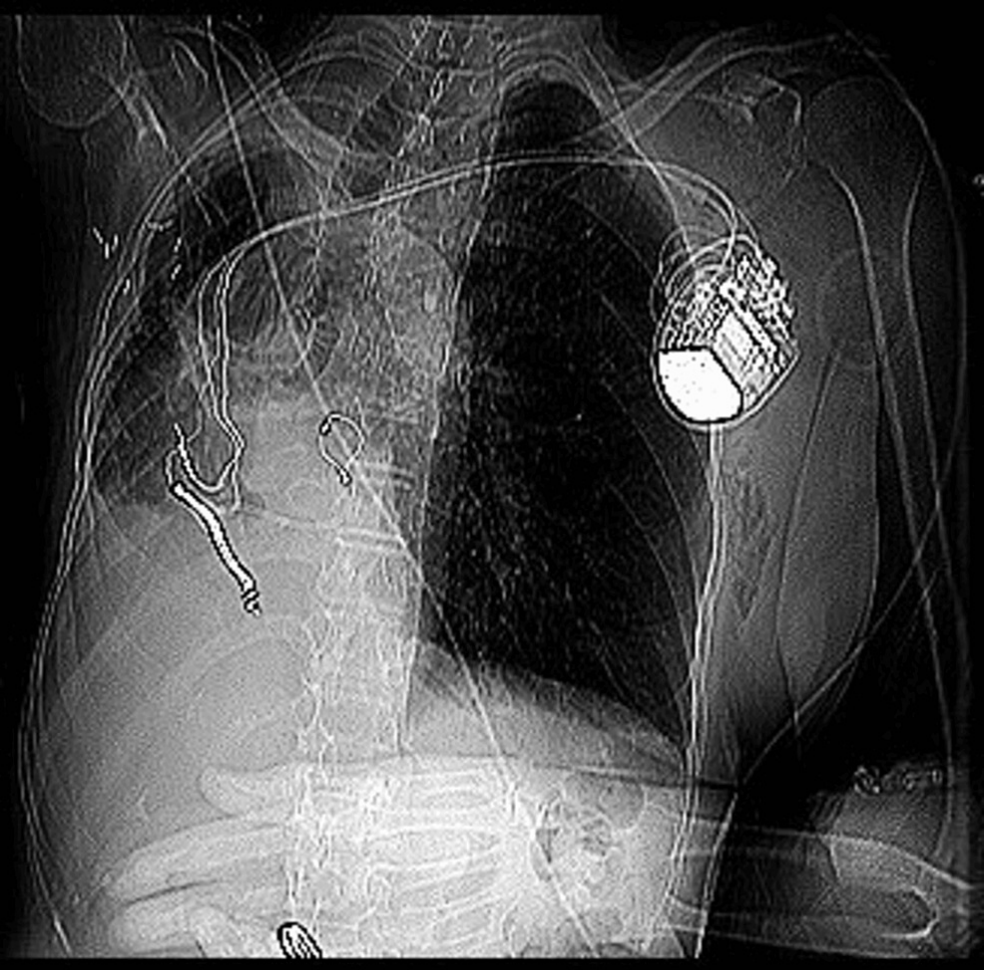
Post-intervention chest CT scan The image shows implantable cardioverter-defibrillator (ICD) leads and pseudo-dextrocardia.
